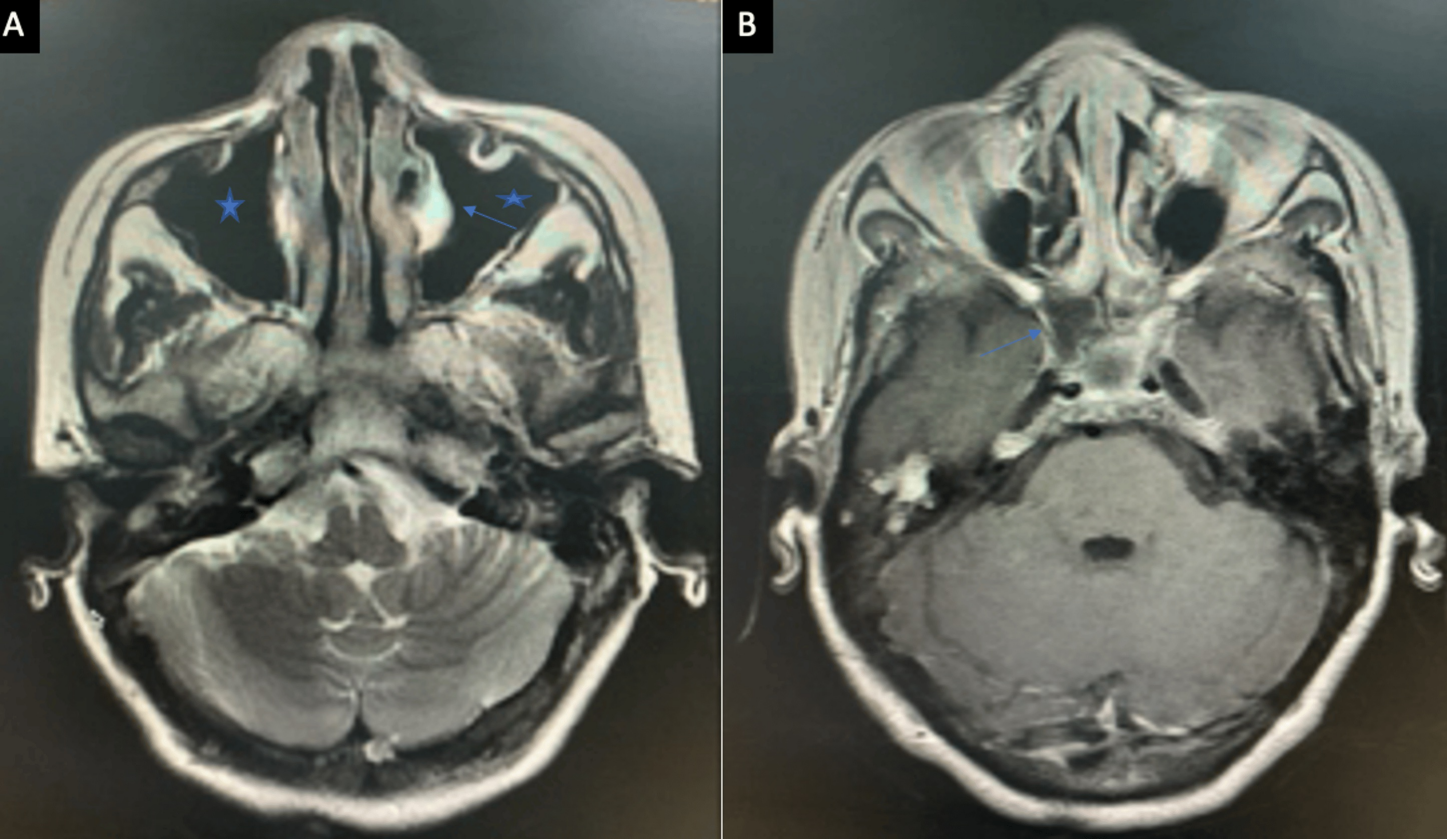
(A) A case of chronic invasive fungal infection showing mucosal thickening in bilateral maxillary sinuses (*) with mucocele in the left maxillary sinus (↗) (MRI, T2 axial). (B) Enhancing soft tissue lesion into right sphenoid sinus and extending up to the cavernous sinus (↗) (MRI, post-contrast T1 axial). MRI: magnetic resonance imaging
